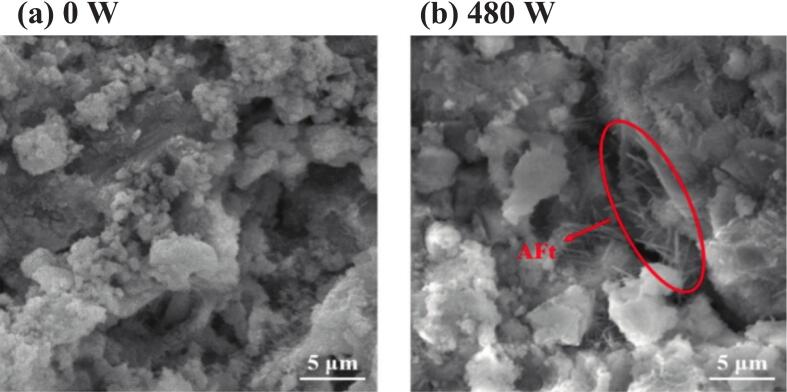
SEM images of samples at different ultrasonic powers.
